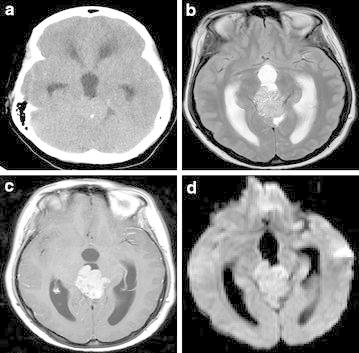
CT scan a shows homogenous mass in pineal region with peripheral calcification and obstructive hydrocephalus. b T2-weighted MRI shows multiple small cysts within the mass and probable invasion of left thalamus, c confirmed on T1-weighted scan after intravenous Gadolinium. d Diffusion-weighted scan (*b* = 1000) shows small area of diffusion restriction anteriorly within the tumour (confirmed on Apparent Diffusion Coefficient map), close to the left thalamus
